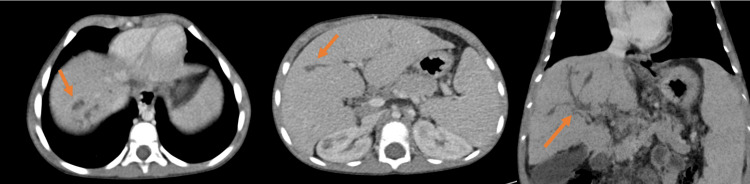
Abdominopelvic CT angiography revealing cystic dilations of the intrahepatic bile ducts in segment VII, measuring 8 mm, along with fusiform peripheral and central dilations communicating with the biliary tree, without any visible obstruction. The liver appears dysmorphic and hypertrophic CT: computed tomography
